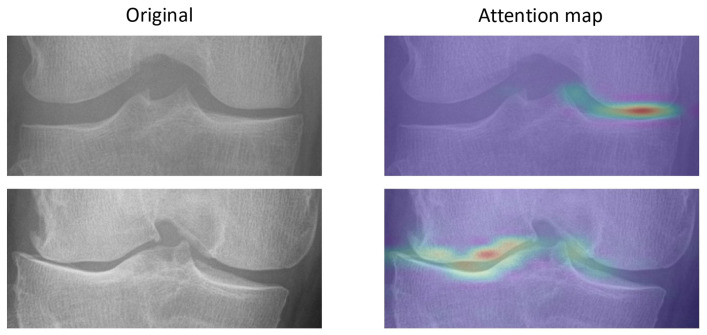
Attention map. The attention map shows that the model can identify OA features, including narrowing of joint space and emerging osteophytes, which are established radiographic markers of progression.
